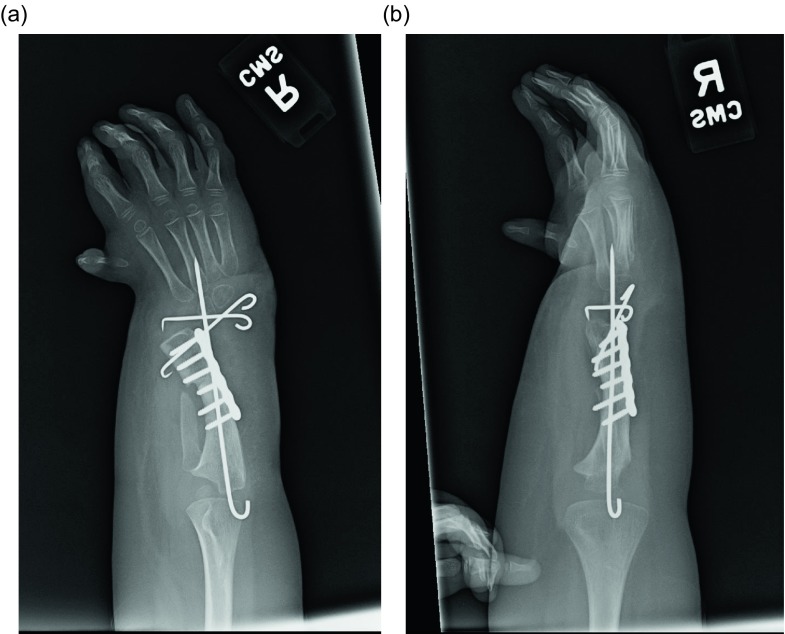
AP and lateral radiographs after ulnarization with shortening. Note the temporary arthrodesis wires in place and the plate on the ulna.
